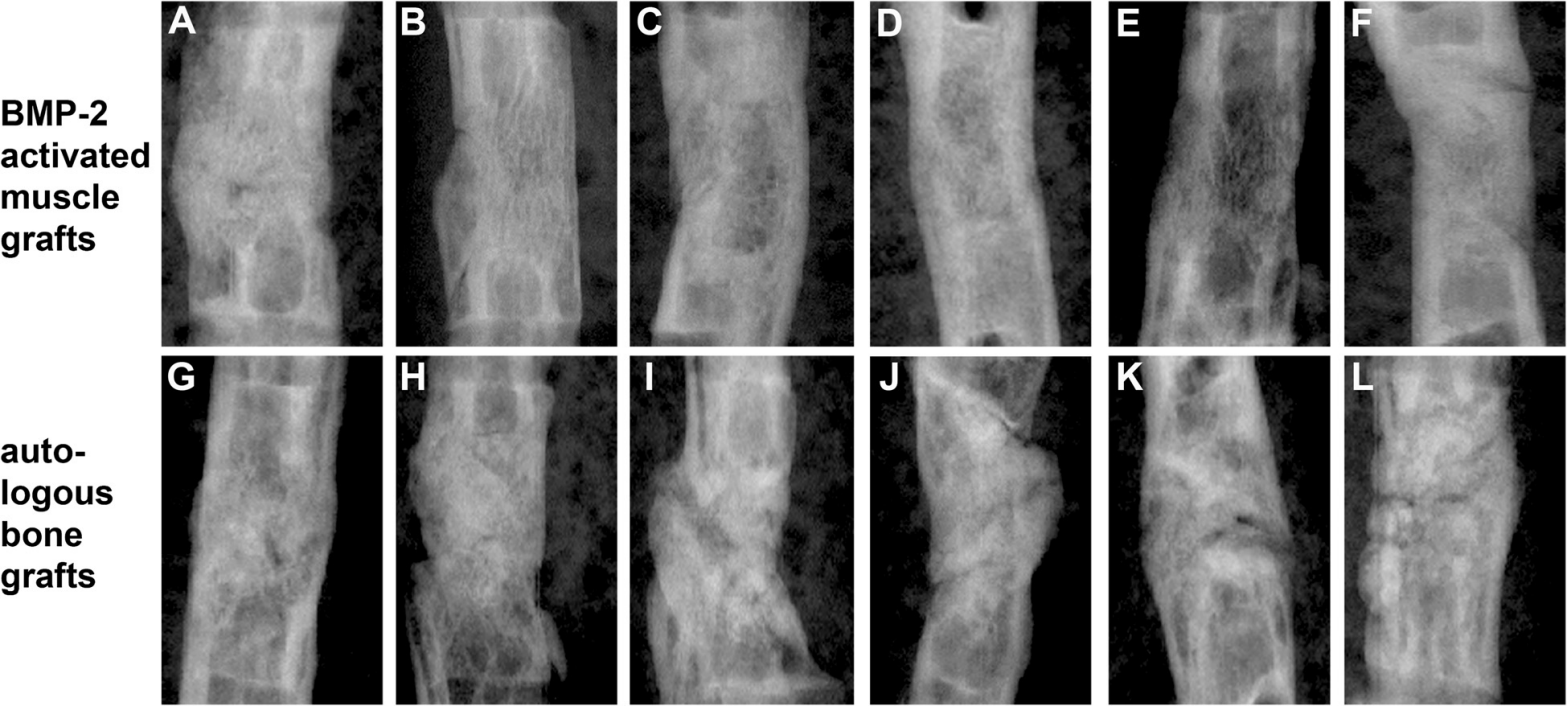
Radiographic images. Radiographic images of femoral segmental bone defects 8 weeks after surgery. Femora treated with BMP-2 gene activated muscle grafts (A - F) and autologous bone grafts (G – L) displayed complete bridging of the defect.
